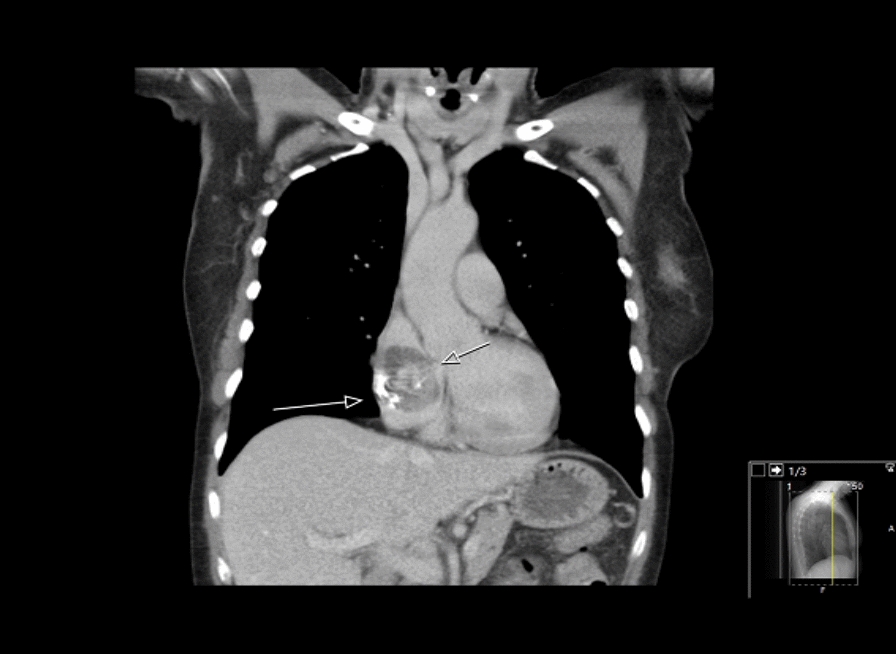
CT-scan of the myxoma in the right atrium
